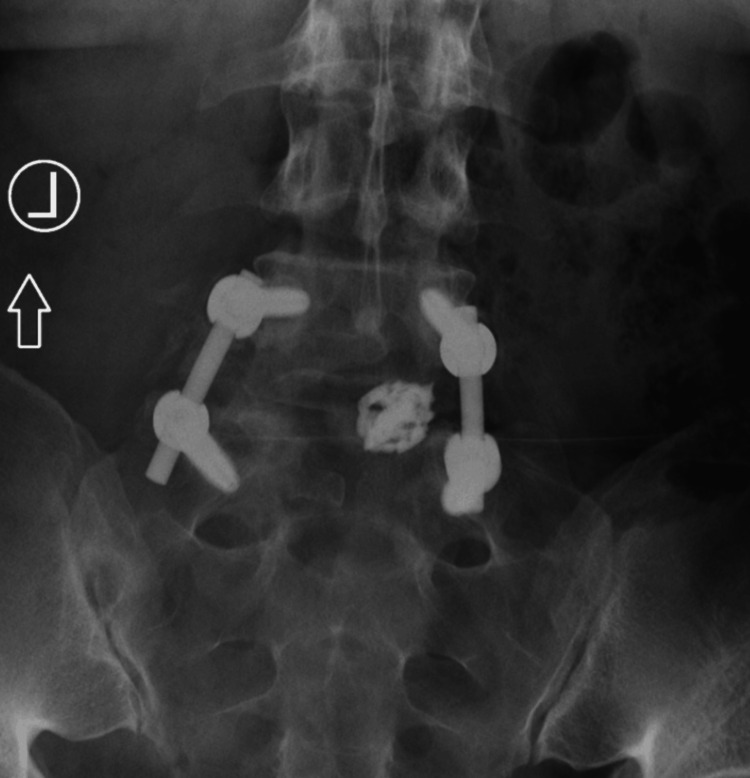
Anteroposterior X-ray of the lumbar spine for Case 1 following the procedure.
